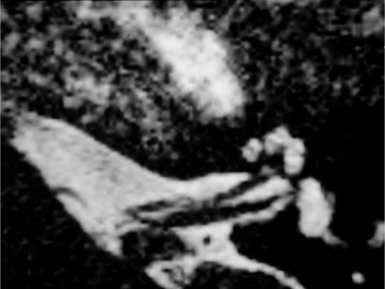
MRI of the temporal bone, T2 image of the cochlea and the internal auditory meatus
